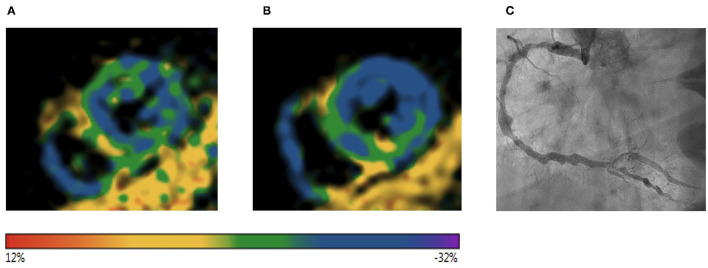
Color-coded fSENC images visualizing longitudinal strain at rest (A) and after DHE (B) of an excluded patient with a positive dobutamine stress test. Invasive coronary angiography revealed a significant stenosis of the right coronary artery (C) (color scale represents regional longitudinal strain). fSENC, fast strain-encoded magnetic resonance imaging; DHE, dynamic handgrip exercise.
